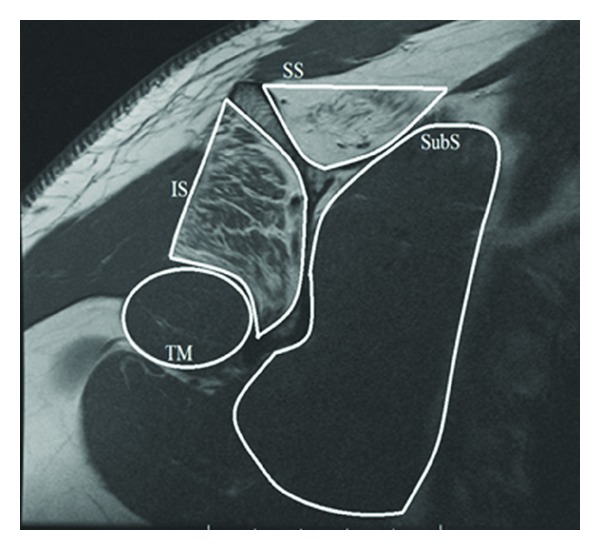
T1 sagittal oblique MRI image of rotator cuff muscles, demonstrating fatty infiltration of the supraspinatus and infraspinatus muscles. IS, infraspinatus; SB, subscapularis; SS, supraspinatus; TM, teres minor.
